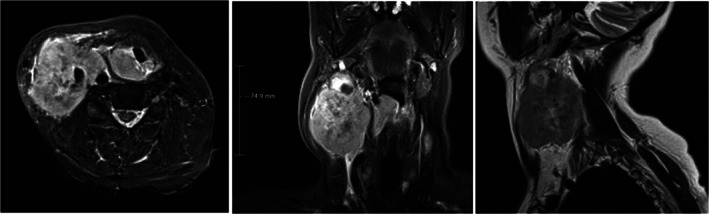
T2‐weighted magnetic resonance imaging head and neck sequences (axial, coronal and sagittal in sequence) showing a 3.5 x 3.4 x 1.6 cm mass centred in the right pyriform fossa, involving the hypopharyngeal surface of the right aryepiglottic fold, extending posteriorly and inferiorly into the hypopharyngeal wall where it crossed the midline. Laryngeal cartilages and larynx itself spared from within. The right level II to IV lymph node mass measured up to 7.5 cm in craniocaudal diameter, encasing the common carotid artery to its bifurcation and obliterating the IJV; the left level III lymph node measured 2.3 cm and level II 1.4 cm
